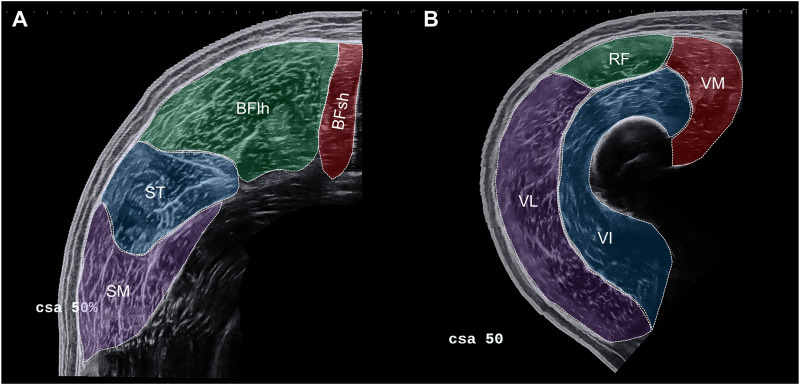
Representative transversal panoramic mode ultrasound images at 50% of the femur length with marked individual hamstrings (A) and quadriceps muscles (B). BFsh: biceps femoris short head, BFlh: biceps femoris long head, ST: semitendinosus, SM: semimembranosus, VL: vastus lateralis, RF: rectus femoris, VM: vastus medialis, VI: vastus intermedius.
